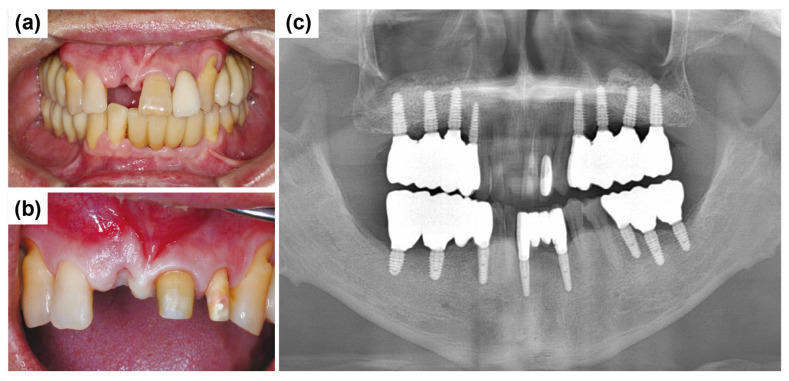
Preoperative clinical and radiographic evaluation. (a) Missing tooth #11 at the initial visit. (b) Preparation of teeth #21 and #22 for fabricating the new restoration. (c) Initial panoramic radiograph shows overall periodontal condition and alveolar bone status.
